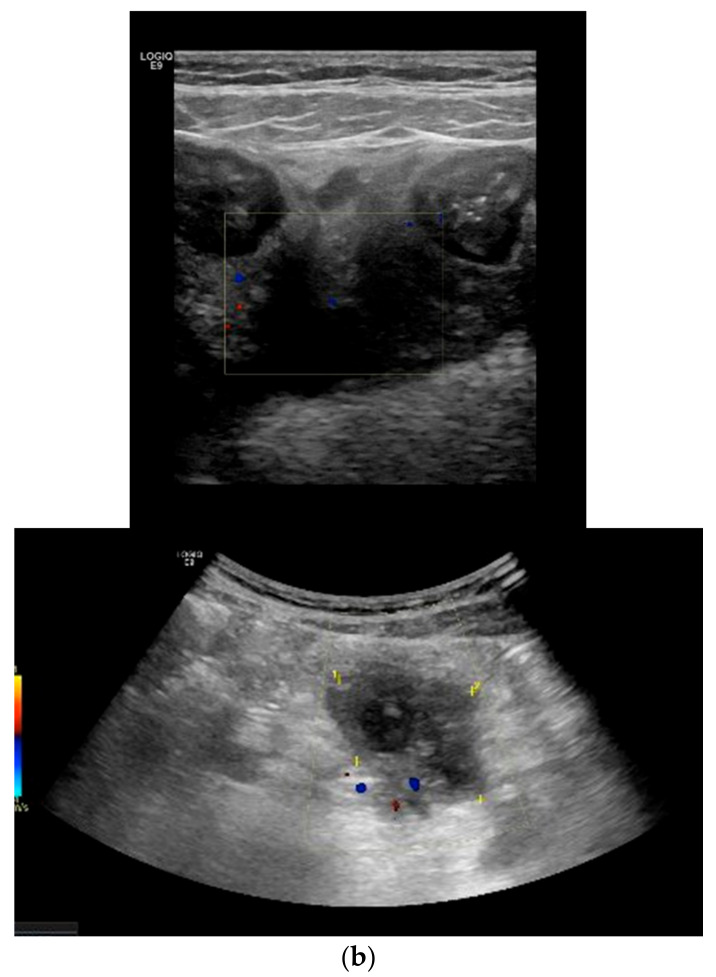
Detection of complications: fistulae and abscesses: (a) The fistulae are seen as hypoechoic peri-intestinal areas with a diameter <2 cm with or without internal gaseous artifacts. (b) Abdominal abscesses are seen as hypo-anechoic lesions containing fluid and gaseous artifacts, posterior enhancement, irregular margins sometimes within fibro-fatty proliferation, without vascular signals in color Doppler.
